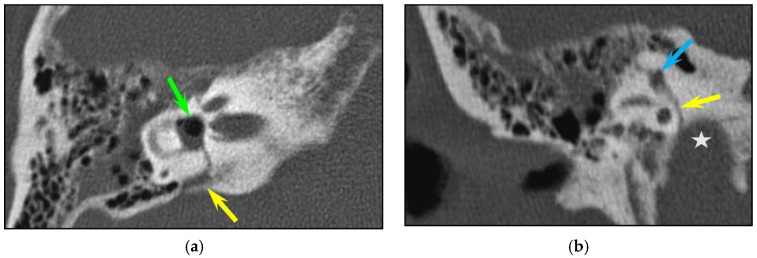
Inner ear injuries. A transverse fracture in an 84-year-old woman with otorrhagia after a fall. CT of the right temporal bone; (a) axial plane; (b) coronal plane. (a) Axial plane: The fracture line extends inferiorly from the aqueduct of the vestibule (yellow arrow) to the vestibule, with associated pneumolabyrinth (green arrow). (b) The fracture line (yellow arrow) also involves the jugular foramen (asterisk) and the superior semicircular canal (blue arrow).
